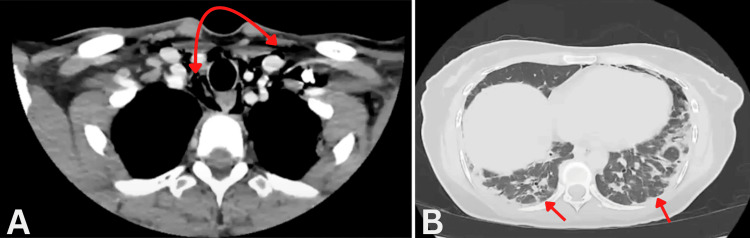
(A) Initial CT showing extensive subcutaneous emphysema throughout the visualized mediastinum and left pharyngeal space (red arrows). (B) Peripherally distributed bibasilar airspace opacities (red arrows) CT: computed tomography
